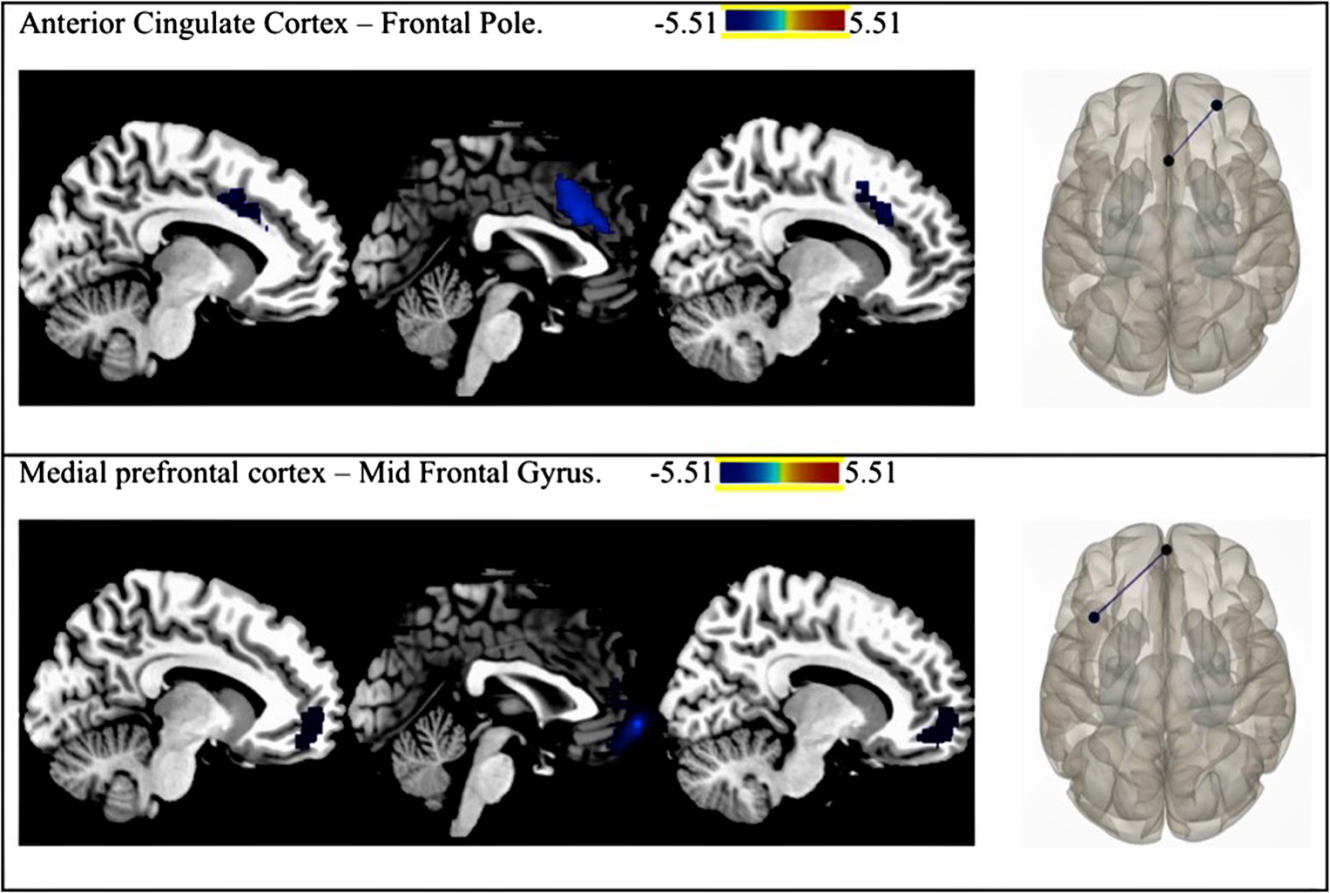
Differences in resting state functional connectivity following a single administration of 2400 mg NAC versus placebo in patients with schizophrenia, in the salience network (top panel) and default mode network (bottom panel). The colour bar represents the *t* value. In the NAC compared to placebo condition, rs-FC was significantly lower between the anterior cingulate cortex (0, 22, 35) and frontal pole (26, 52, 8) and between the medial prefrontal cortex (1, 55, − 3) and mid frontal gyrus (− 38, 18, 42) (*p <* 0.05, FDR corrected). Results are overlaid on sagittal T1-weighted MRI images (left) (Holmes et al. 1998; Rorden et al. 2007), and complementary axial images generated in CONN (right) display the connectivity between the seed and target ROIs.
